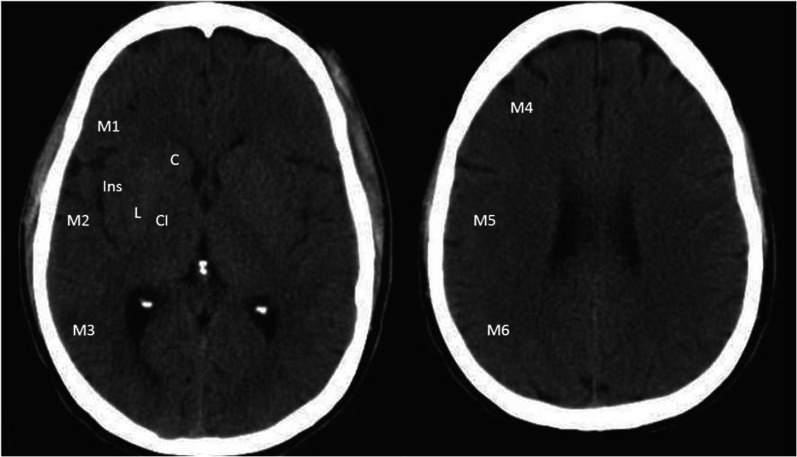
ASPECTS areas: Insula (I), Lenticular (L), Caudate (C), Capsule Internal (CI) and cortical areas: M1, M2, M3, M4, M5 and M6
